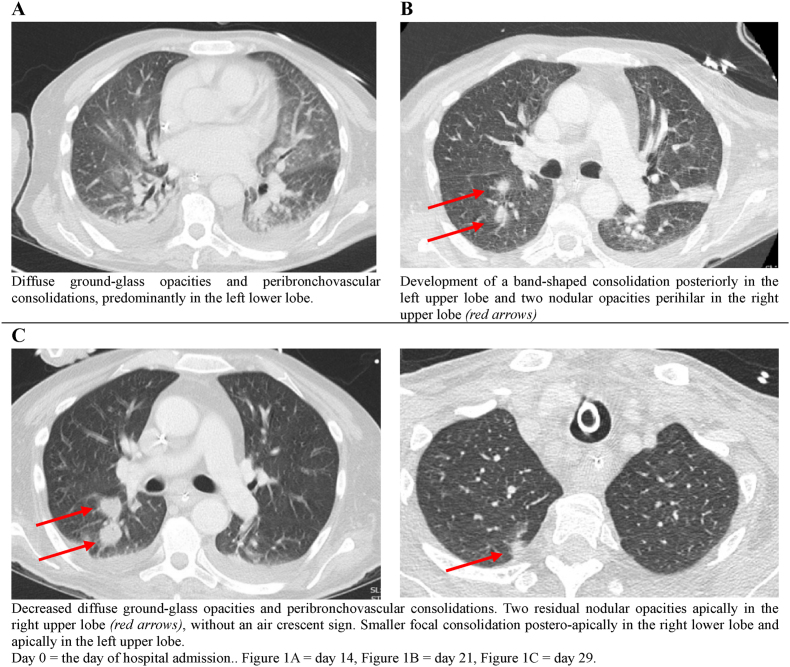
Radiological signs on chest CT.
